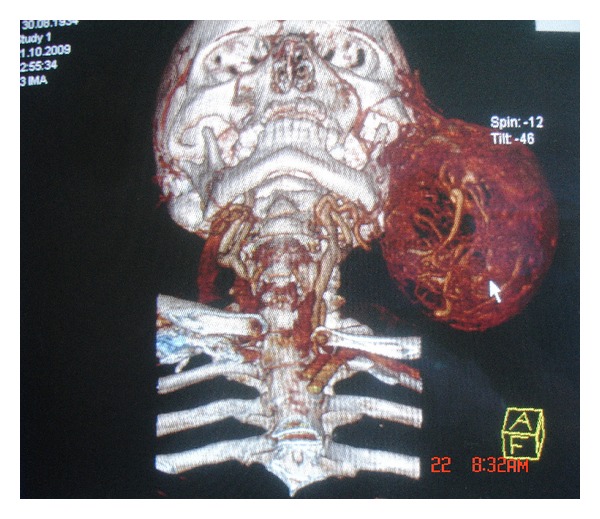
CT angiography displaying the rich vascular network.
